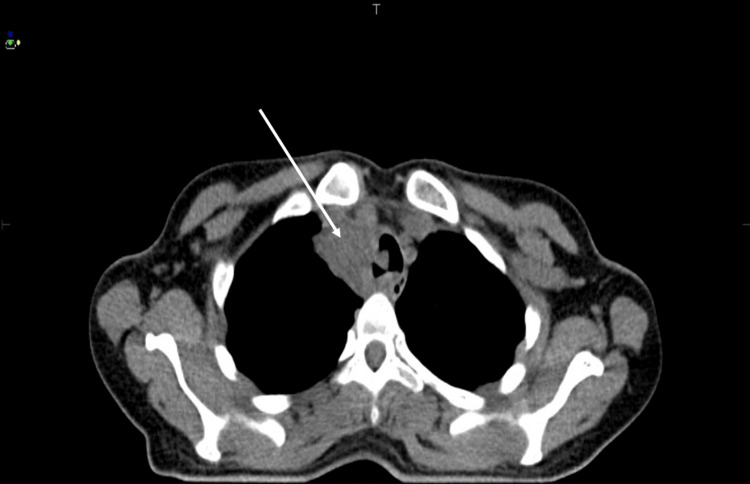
Axial CT image of the trachea showing the upper extent of the lesion. CT, computed tomography.
